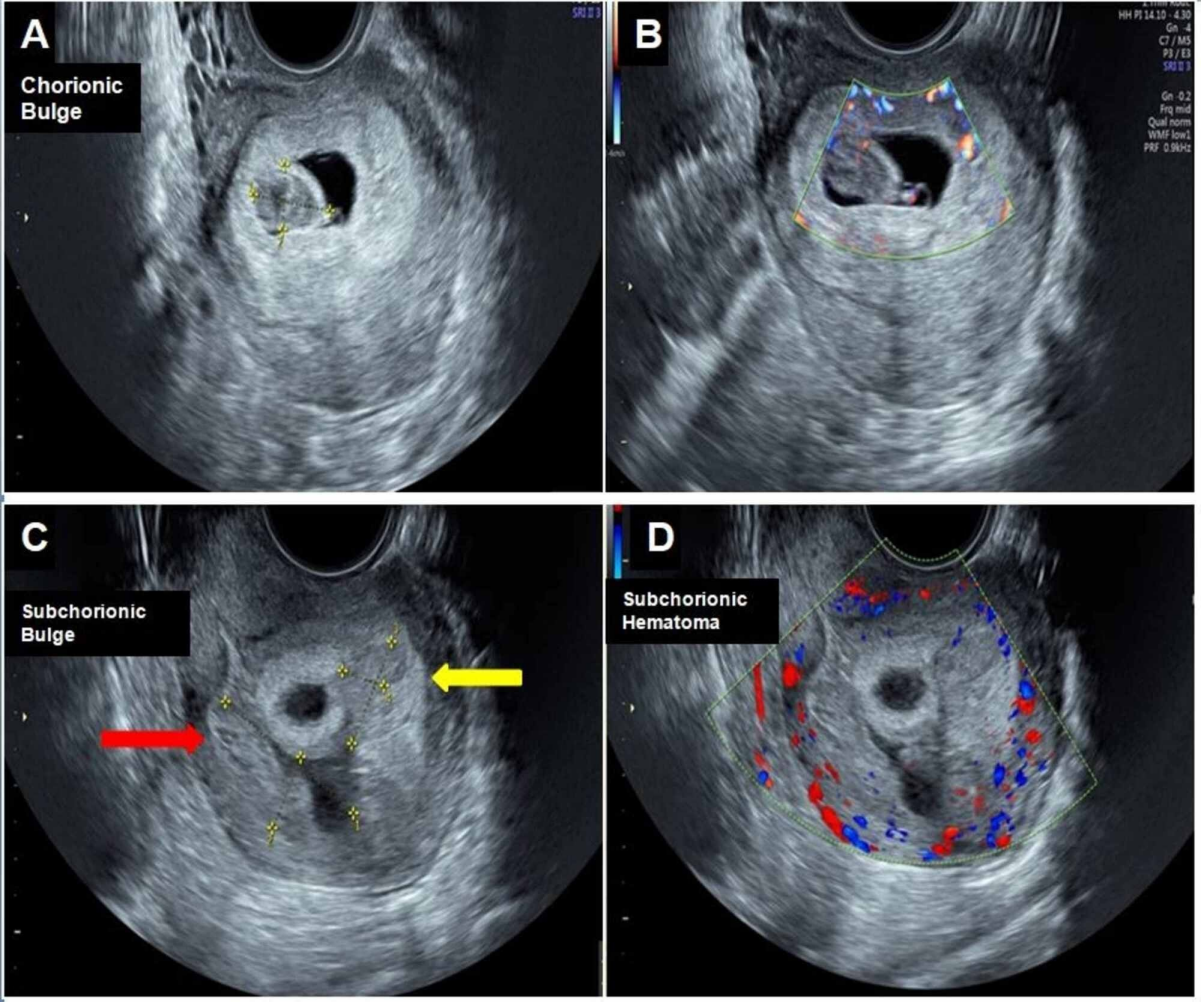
Transvaginal ultrasound (A & B) An oval-shaped bulging lesion from the chorionic wall into the gestational sac, with the hypoechoic center and hyperechoic (isoechoic to chorion) rim measuring 15 X 18 mm in maximum dimensions and showing no blood flow on color Doppler, which is consistent with a chorionic bump (hematoma). (C&D) Two heterogeneous lesions can be seen in the subchorionic area, measuring 2.8 x 1.1 cm (red arrow) and 1.5 x 0.8 cm (yellow arrow), demonstrating no internal flow on color Doppler; they are subchorionic hematomas.
